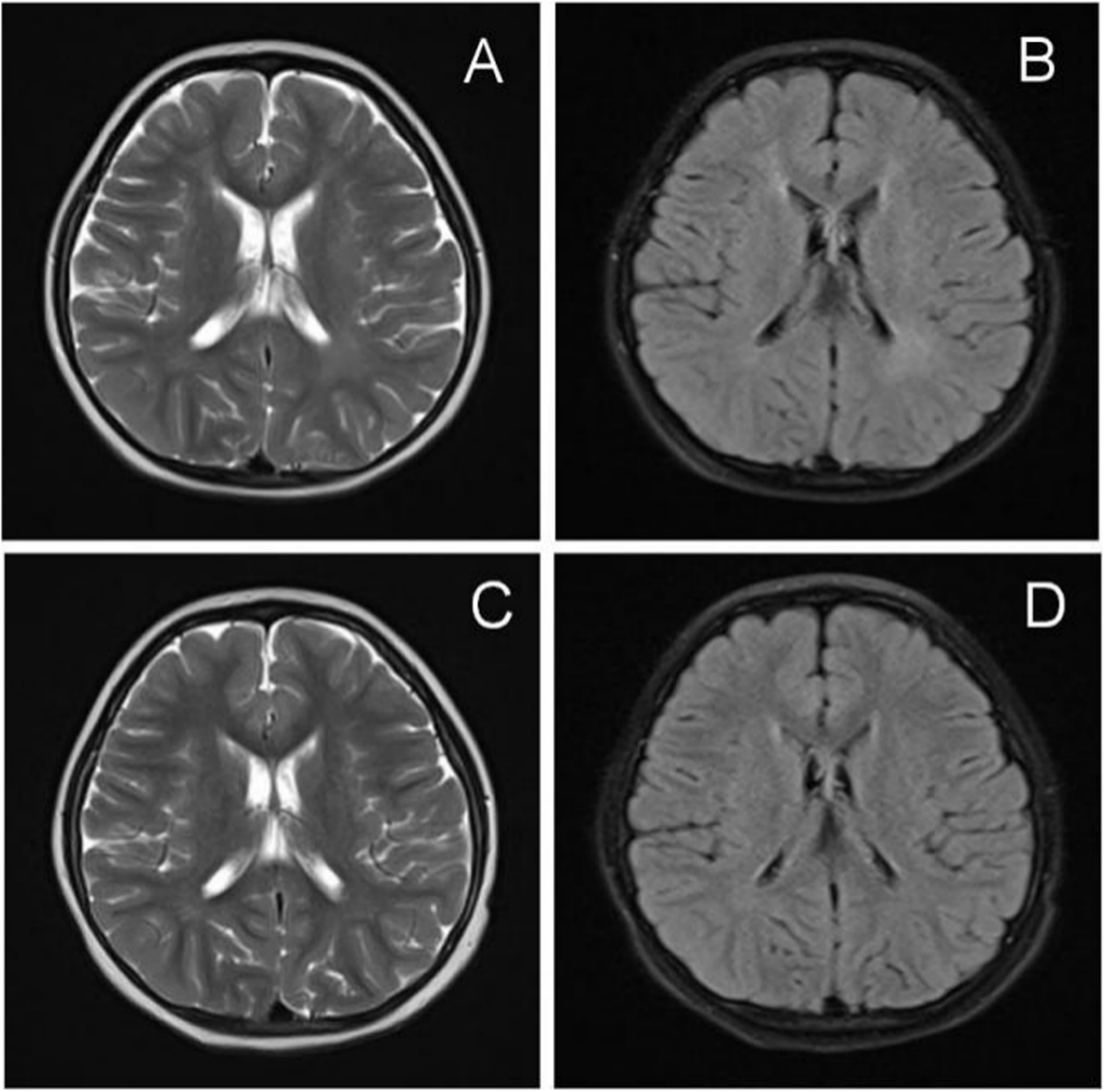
MRI results of 4-and 5-year-old heads of children. Images taken by A. T2WI, B. Flair, C. T2WI, D. Flair, T2WI and Flair showed that the areas of high signal intensity in bilateral lateral ventricles and patchy areas in bilateral frontal lobes were smaller than those at the time of admission
